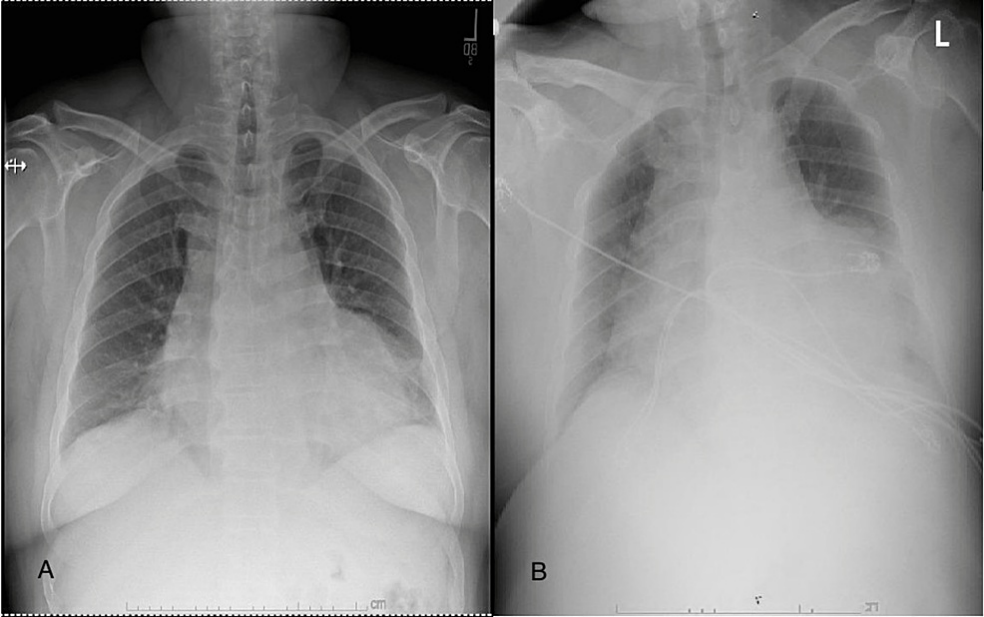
Chest X-Ray Initial chest x-ray (A) as compared to chest x-ray obtained at the onset of hemodynamic instability (B) showing increased cardiac silhouette.
